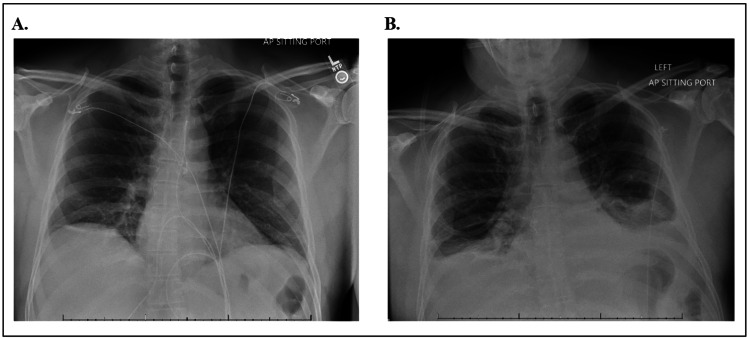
A. AP chest X-ray shows no pleural effusion on day 1 of hospitalization; B. AP chest X-ray shows mild to moderate pulmonary interstitial edema, suggesting pneumonia on day 4 of hospitalization
